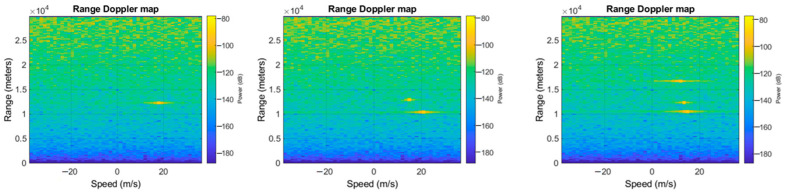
Example of range-Doppler maps generated with speeds of 225 m/s and different positions of (respectively, from the left to right) 1, 2, 3 targets.
